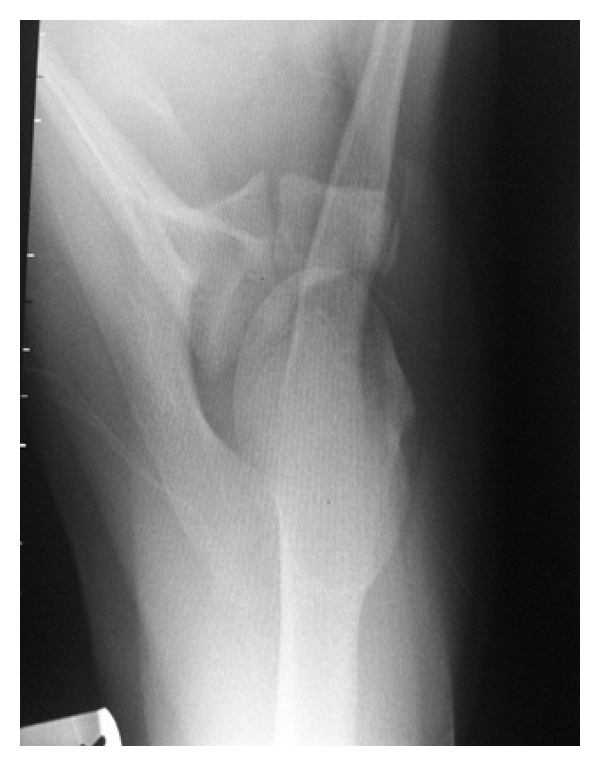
Case B: initial axillary radiograph.
